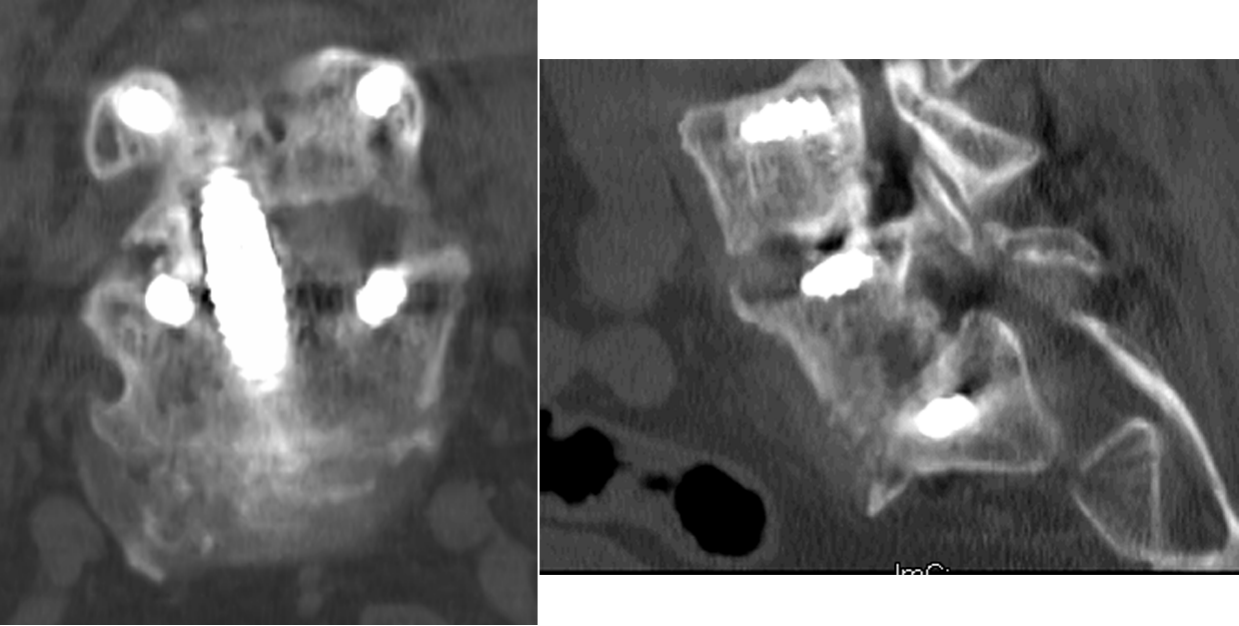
Computed tomographic coronal (left) and sagittal (right) images demonstrate successful fusion at one-year follow-up.
